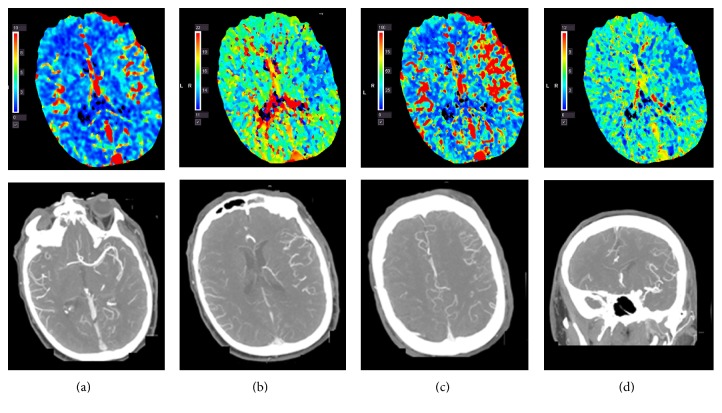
Hyperperfusion on computed tomography perfusion and the dilation of the left middle cerebral artery and its branches on CT angiogram after iv tPA treatment: (a) increased cerebral blood volume; (b) shortened time to peak; (c) increased cerebral blood flow; (d) shortened mean transit time.
